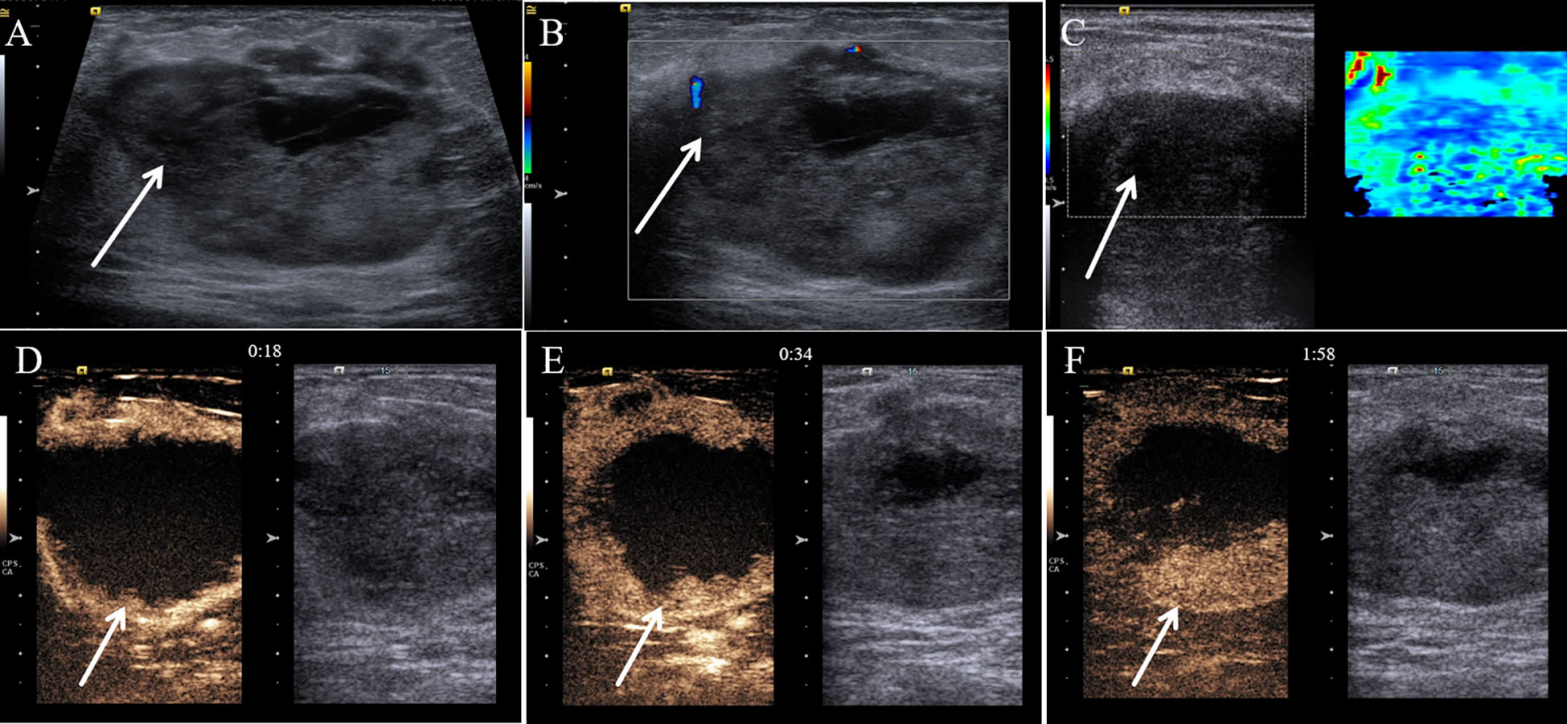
Multimodal ultrasound performance of malignant glomus tumor in the breast. (A) Conventional gray-scale sonography revealed a mixed solid and cystic lump (arrow) in the breast. (B) Color Doppler flow imaging showed several blood flow signals that were observed in the periphery of the tumor (arrow). (C) Shear wave elastography showed a soft nodule (arrow) of the breast. (D) The contrast-enhanced ultrasound image captured 18 s after the injection of the contrast agent. (E) The contrast-enhanced ultrasound image captured 34 s after the injection of the contrast agent. (F) The contrast-enhanced ultrasound image captured 118 s after the injection of the contrast agent.
